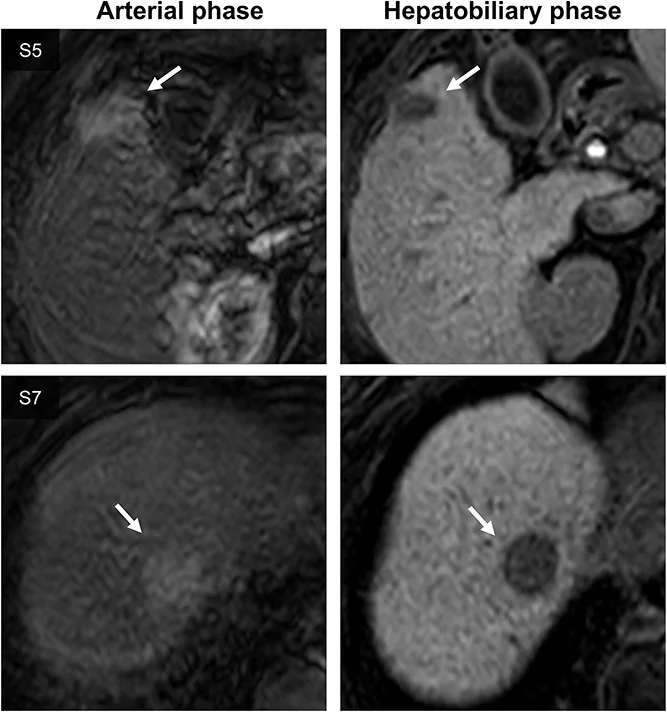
Baseline EOB-MRI findings of hepatocellular carcinoma tumors in segments 5 and 7. Arterial-phase and hepatobiliary-phase images are shown. The arrows indicate the lesions.
